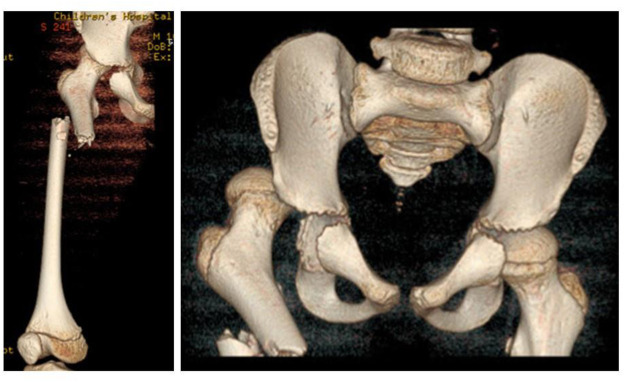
Computed tomography(CT) showed that a displaced fracture of the proximal-middle segment of the right femoral shaft and a posterior dislocation of the right hip joint.
